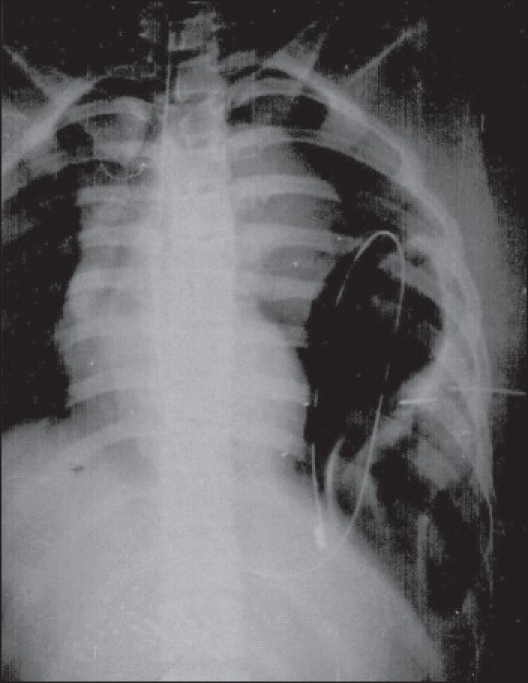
X-ray chest showing intercostal tube and Ryle's tube lying in the left hemithorax
